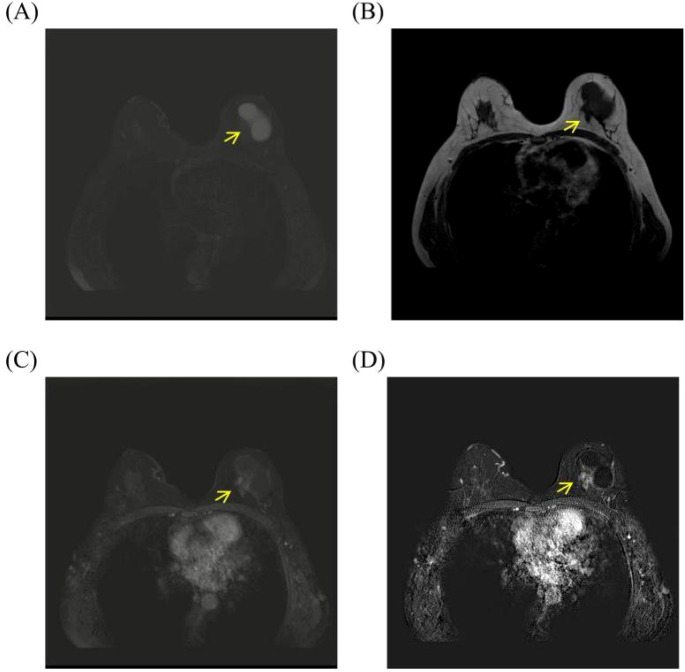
MRI findings of Case 2. (A) Axial T2-weighted SPAIR image shows a round cystic and solid mass in the left breast. The cystic component demonstrates marked hyperintensity, whereas the mural solid component shows intermediate signal intensity with smooth, well-circumscribed margins. (B) Axial T1-weighted TSE pre-contrast image shows the lesion to be isointense to slightly hypointense relative to the surrounding fibroglandular tissue. (C) Early dynamic contrast-enhanced eTHRIVE image demonstrates mild, relatively homogeneous enhancement of the intracystic solid component without irregular margins, spiculation, or adjacent invasive features. (D) Subtraction image confirms true enhancement of the mural solid component while suppressing background parenchymal signal, allowing clearer delineation of the enhancing portion of the lesion.
